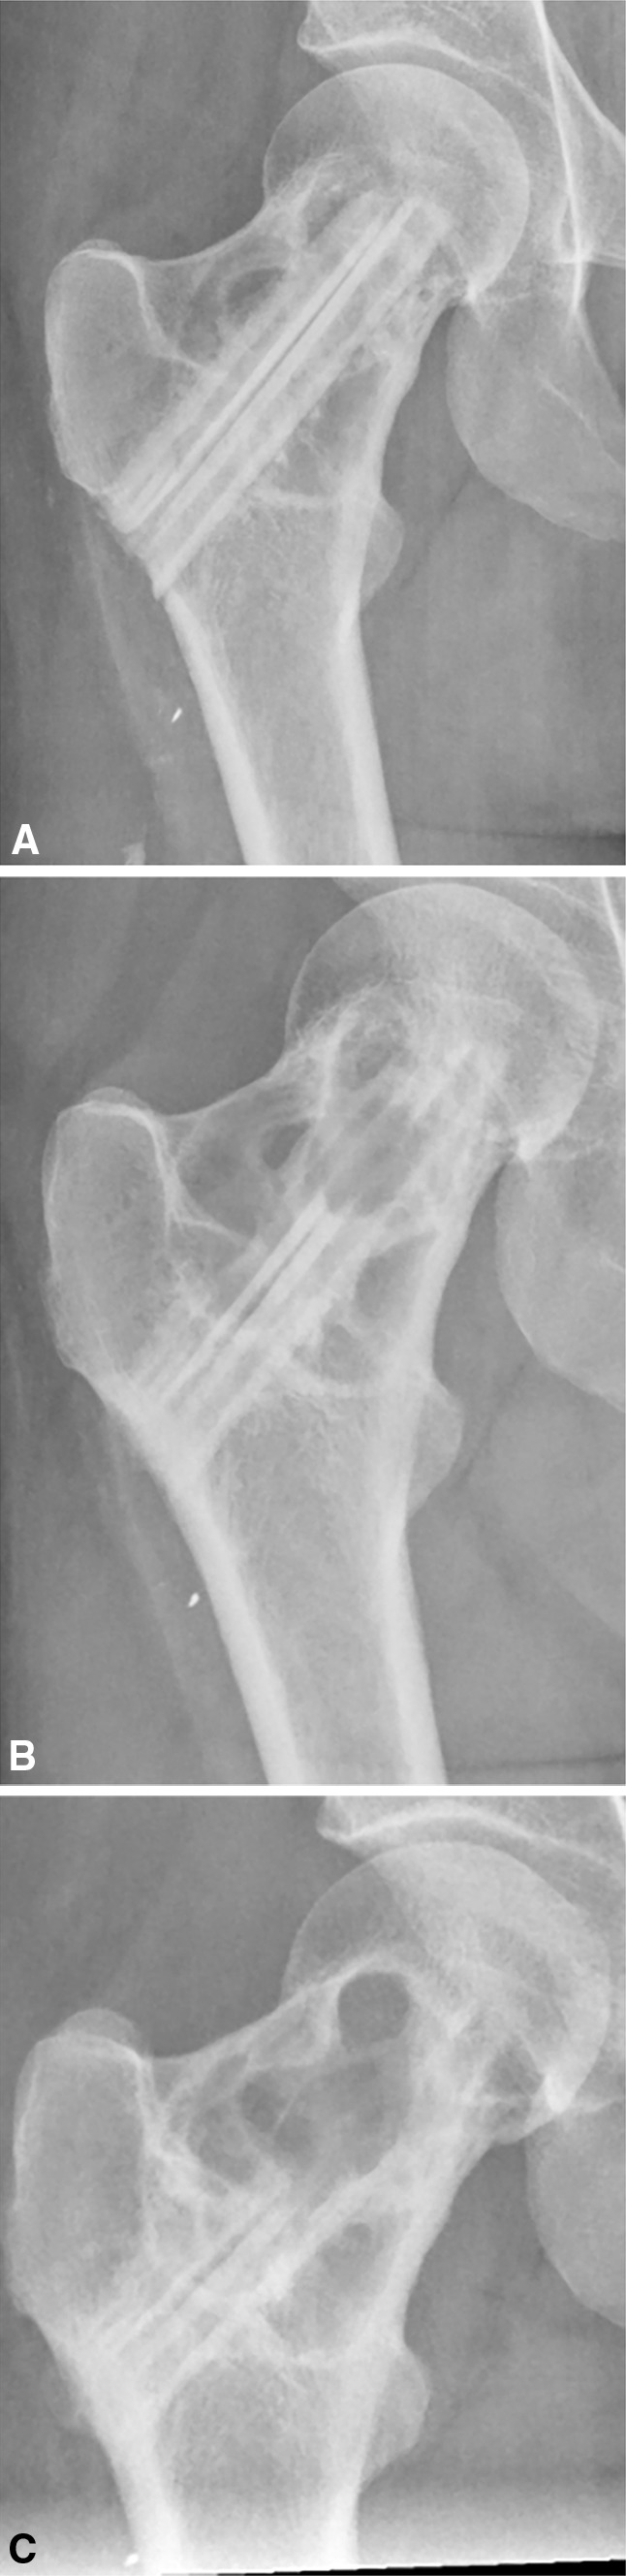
A–C The radiograph (A) is made postoperatively and shows two fibular strut grafts that cross the dysplastic lesion but have minimal contact with vital bone proximally. After 1 year, resorption of the graft gradually increased (B) and finally the strut graft is resorbed over the full length of the diameter (C), losing its stabilizing function.
